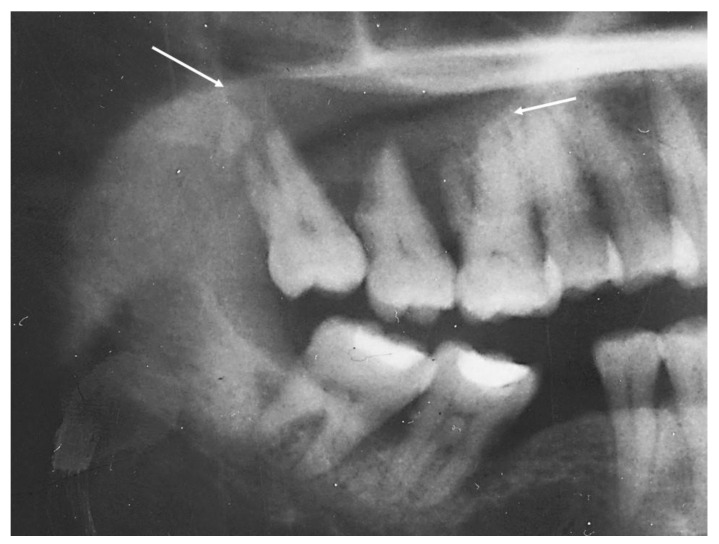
Panoramic X-ray film revealing poorly demarcated radiolucency in the right posterior maxilla.
